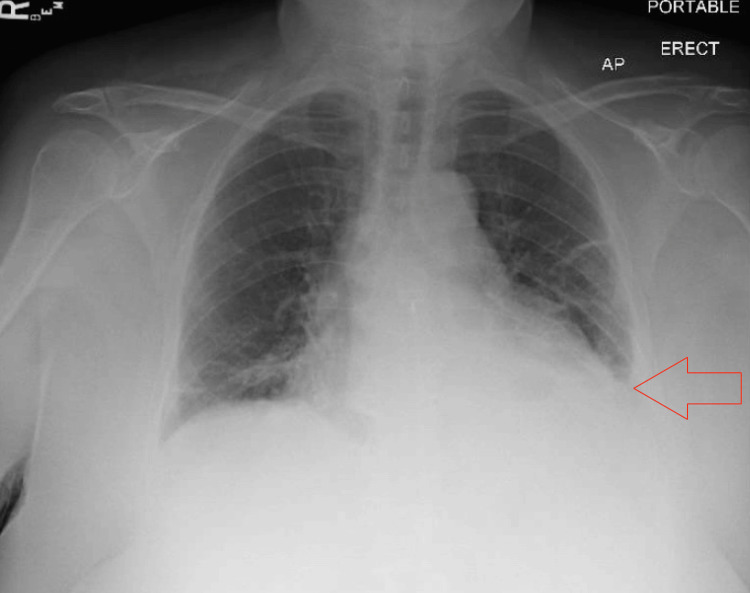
Portable chest radiograph demonstrating a small left pleural effusion (red arrow) and bilateral linear opacities consistent with atelectatic change.
